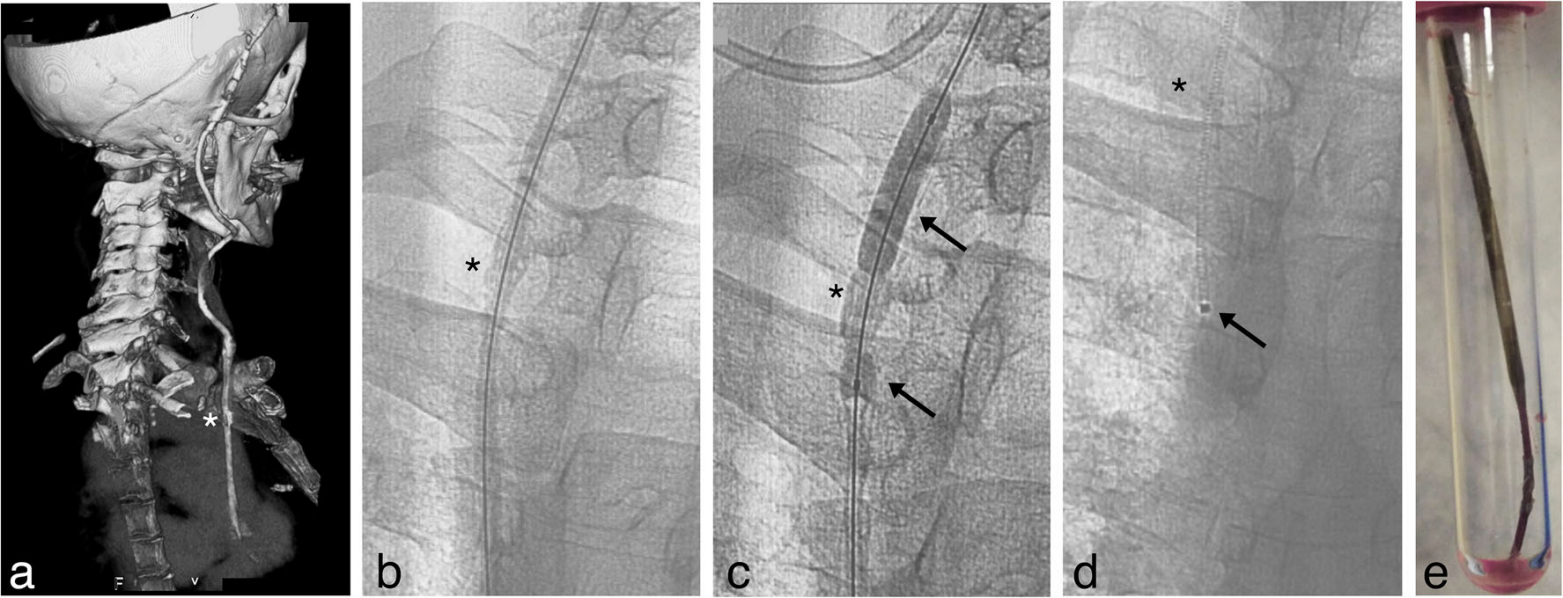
Removal of VAS catheter in patient 1. a Preoperative 3D CT scan reconstruction of the VAS catheter path from the valve to the right atrium, white asterisk (*) marks the site of macroscopic calcifications surrounding the catheter. b Fluoroscopy antero-posterior projection, the 0,018 inch guidewire is visible inside the VAS catheter, black asterisk (*) marks the site of the same macroscopic calcification shown in Fig. 1a. c Fluoroscopy antero-posterior projection, the VAS catheter was dilated by a 6 mm diameter Sterling balloon catheter. The calcification surrounding the VAS catheter partially restricts the dilation of the lumen compared to the adjacent segments (arrows), asterisk as in Fig. 1b. d Fluoroscopy antero-posterior projection, obtained after complete removal of the VAS catheter arrow points to an angiography catheter introduced after the VAS catheter was completely removed. e Picture of the distal portion of the VAS catheter immediately after extraction, the catheter is encrusted by calcified reactive tissue and partially deformed
